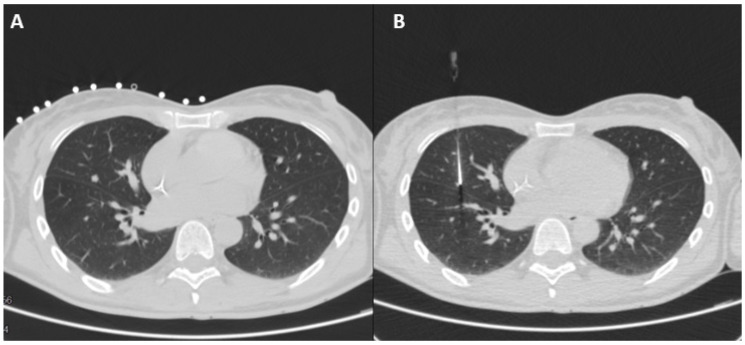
Preoperative CT-guided procedure: (A) localizer-computed tomography to detect the pulmonary nodule; (B) CT-guided percutaneous injection of indocyanine green.
